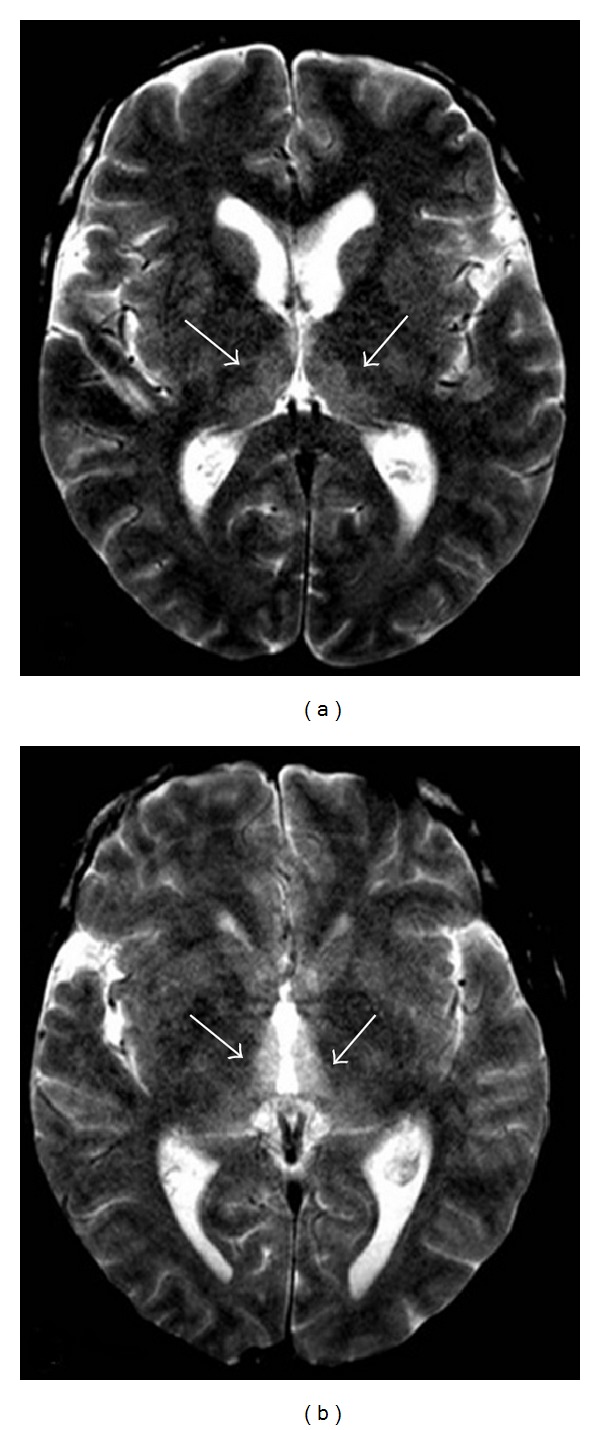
Forty-three-year-old man with a 10-year history of alcohol abuse. T2-weighted axial image showing bilateral and symmetric hyperintense signal alteration at the level of the medial portion of the thalami.
